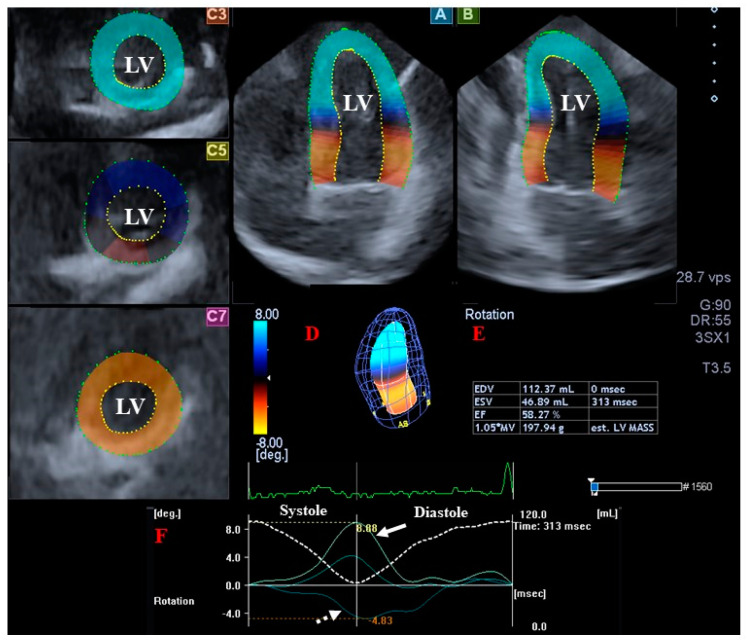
Assessment of the left ventricle (LV) by three-dimensional (3D) speckle-tracking echocardiography. Apical four-chamber view (A); apical two-chamber view (B); and short-axis views at basal (C3), midventricular (C5), and apical LV levels (C7) are presented together with a 3D LV cast (D) and calculated LV volumetric data (E). The LV apical rotation curve (white arrow) and LV basal rotation curve (dashed white arrow) are also presented, together with the LV volume change curve during the cardiac cycle (dashed white line) (F). Abbreviations: LA, left atrium; LV, left ventricle; RA, right atrium; RV, right ventricle; EDV, end-diastolic volume; ESV, end-systolic volume; EF, ejection fraction.
